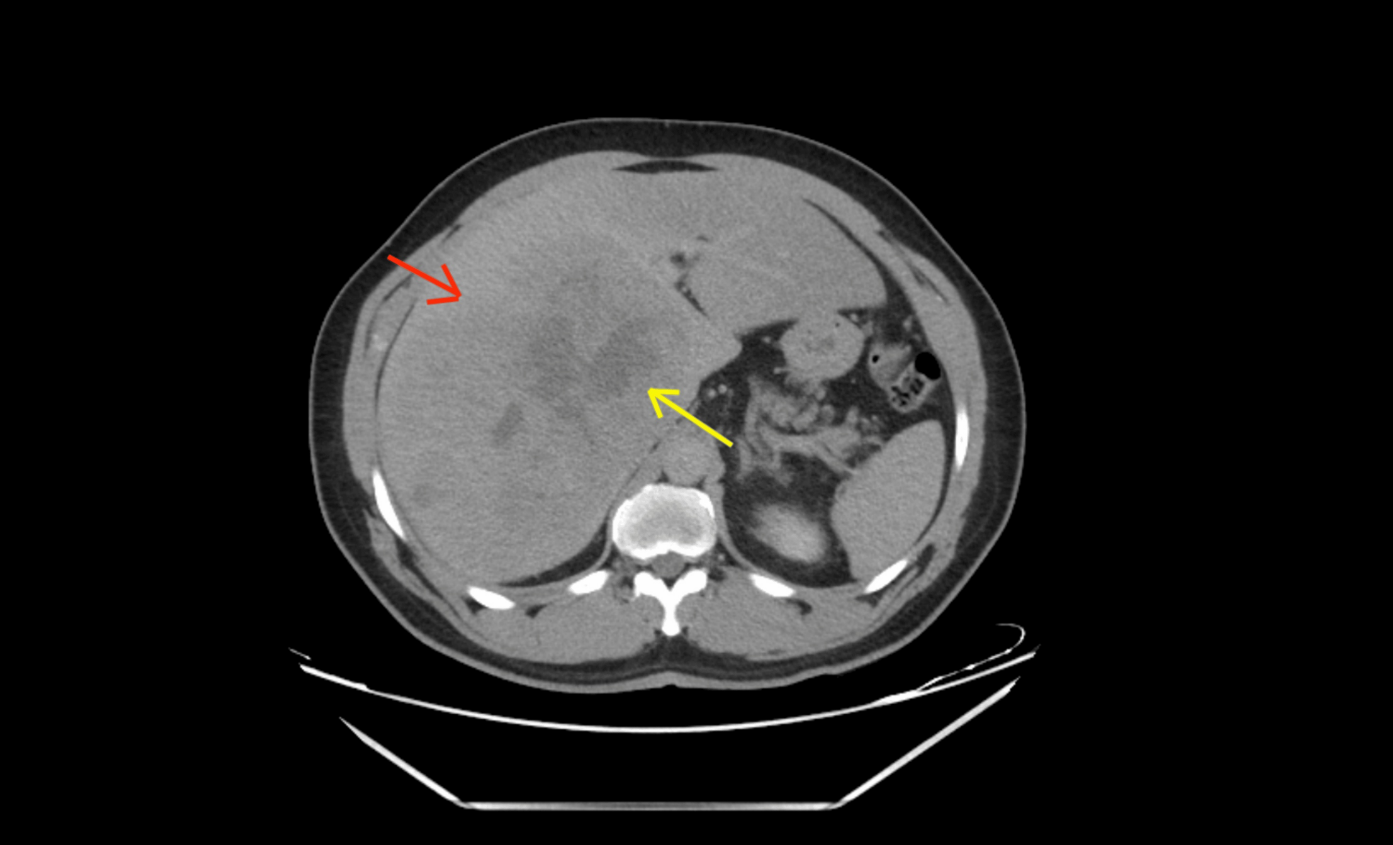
Triple-phase CT with liver mass protocol (delayed phase) Seen is a wash of the enhancement of the mass and the capsule around the mass (red arrow) and further hypodensity of the mass (yellow arrow). This is evident as the rest of the liver is getting more blood supply containing the contrast from the portal vein compared to the mass, which is receiving its supply from the hepatic artery.
